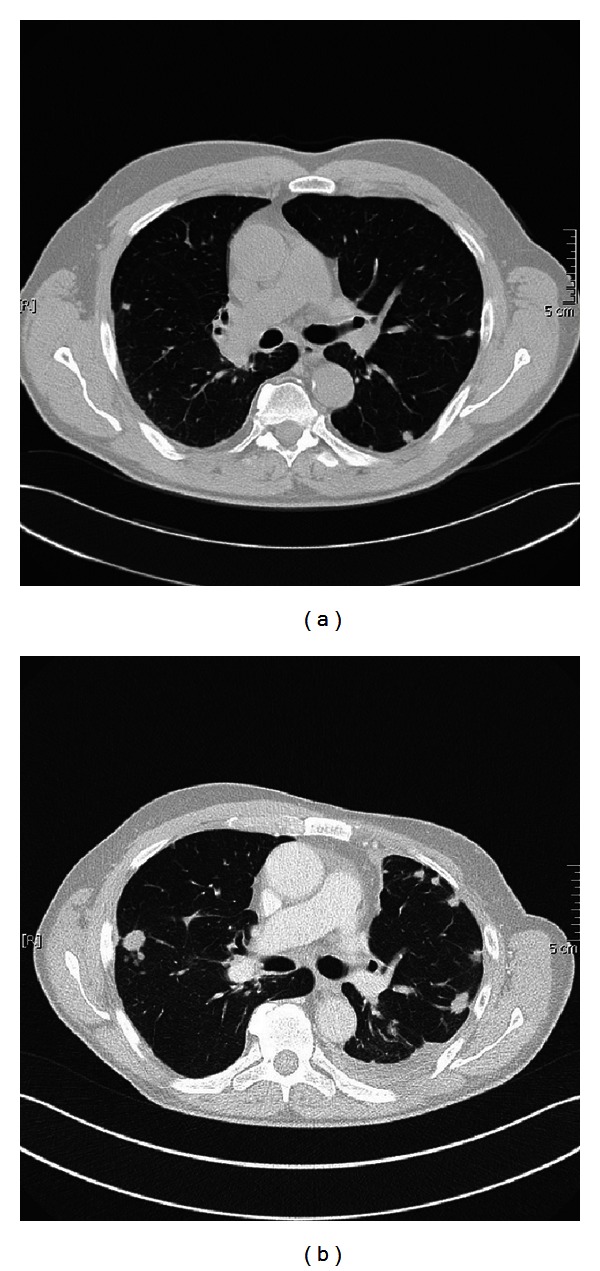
CT scan before the palliative chemotherapy with docetaxel and gemcitabine (a); the reevaluation CT scan (b) showed clear bilateral pulmonary progression after multiple cycles of palliative chemotherapies.
